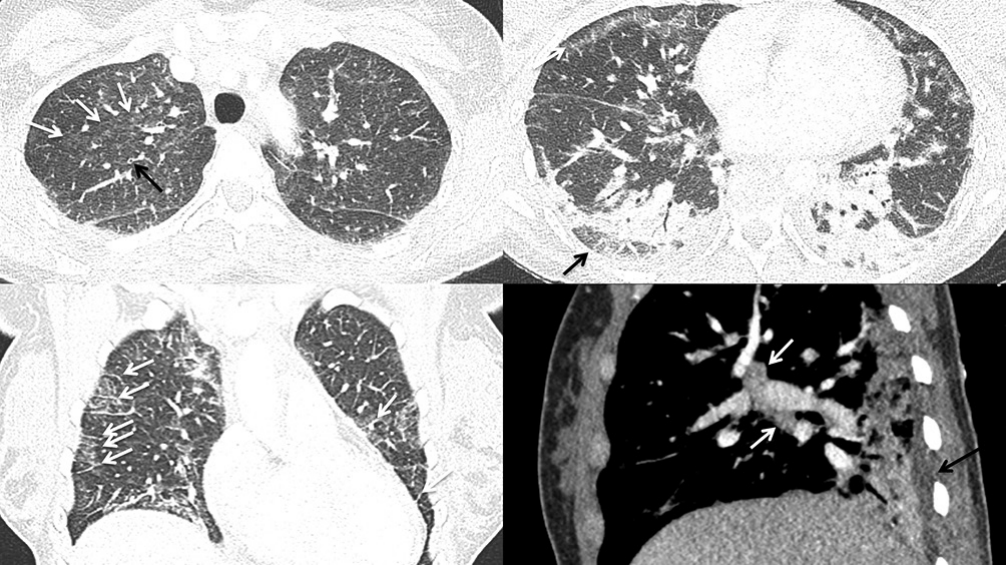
A (top left). Axial HRCT image showing centrilobular groundglass opacities (white arrows) and bronchial wall thickening (black arrow) in the upper lobes B (top right). Axial HRCT showing showing groundglass opacities with subpleural sparing (white arrow) in the right middle lobe and lingula and peribronchovascular and peripheral consolidation in the lower lobes with subpleural sparing (black arrow) C (bottom left). Coronal CT image showing groundglass opacities and septal thickening (white arrows) D (bottom right). Sagital CT image showing mild hilar lymphadenopathy (white arrows) and trace pleural effusions (black arrow). HRCT, high-resolution CT
